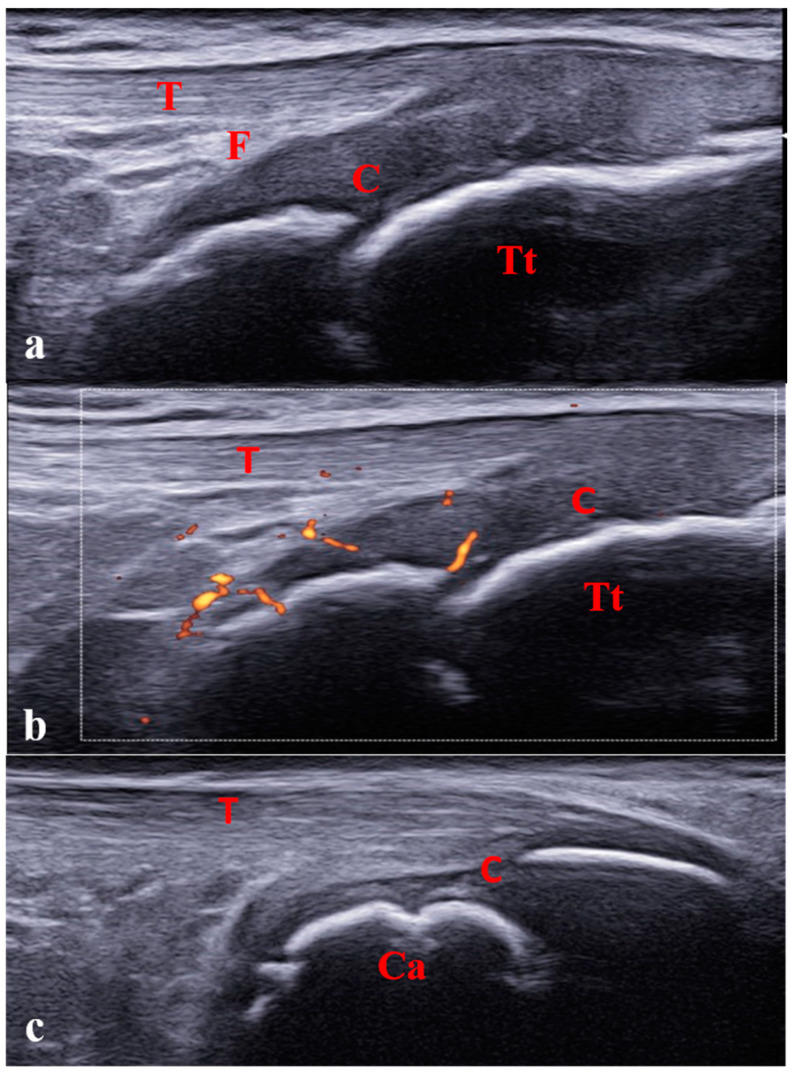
Pediatric ultrasound peculiarities. Increased ratio of cartilage to bone (a) and physiological cartilage vascularization (b) in a 6-year-old girl. (c) Physiological irregularity of calcaneus bone surface owing to the presence of ossification centers in a 10-year-old boy. C: cartilage; Tt: tibial tuberosity; Ca: calcaneus; T: tendon; F: fat.
